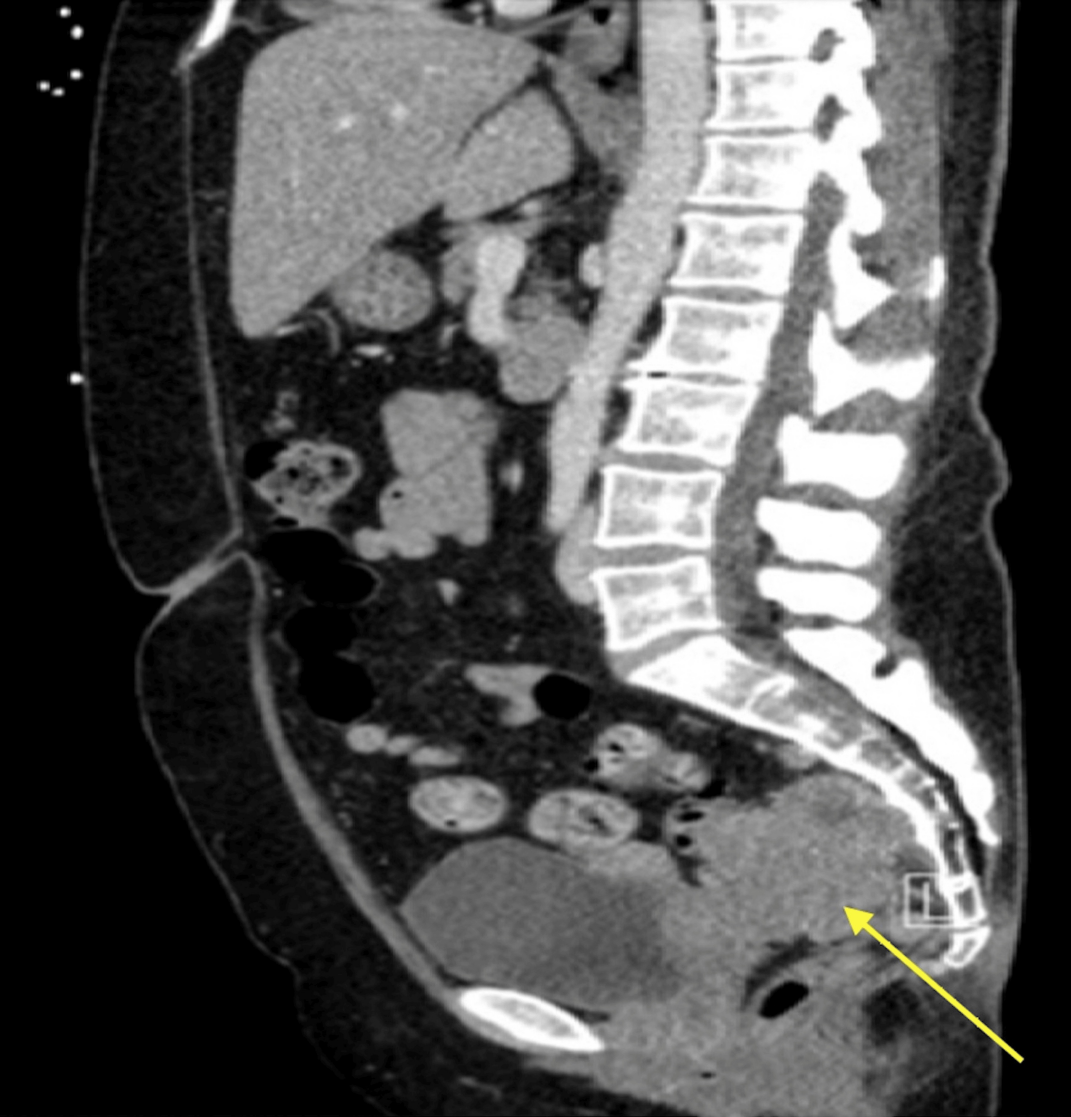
Sagittal CT showing heterogeneously enhancing presacral, posterior lower uterine soft tissue mass (yellow arrow) measuring 6.7 x 5.0 x 6.2 cm.
